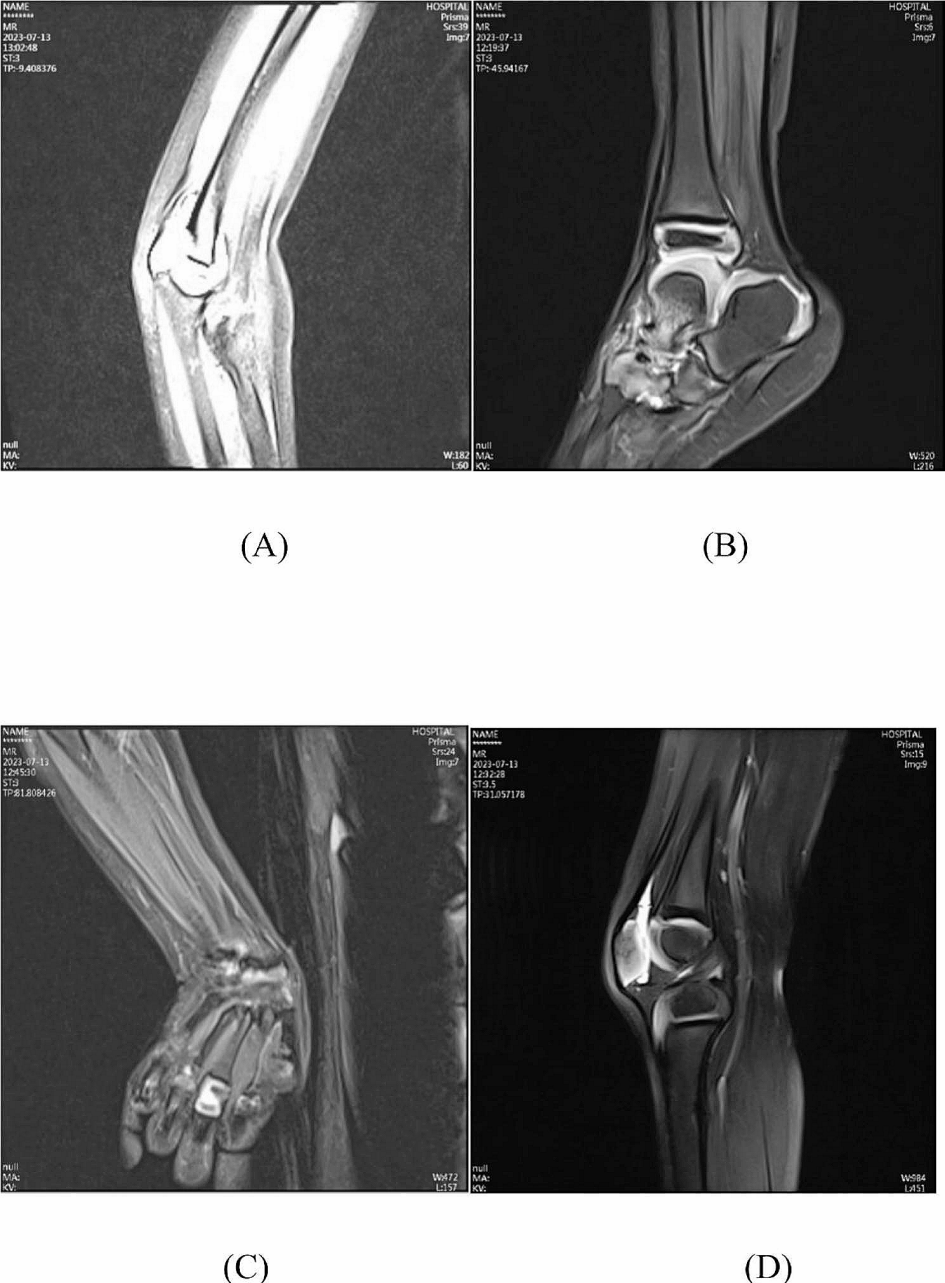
MRI showed decreased articular effusion of the right elbow (A) and no deterioration of destruction and bone resorptions of the ankle joint (B), metatarsophalangeal joints and interdigital joints (C), and left knee joint (D) after 1 more year of treatment
